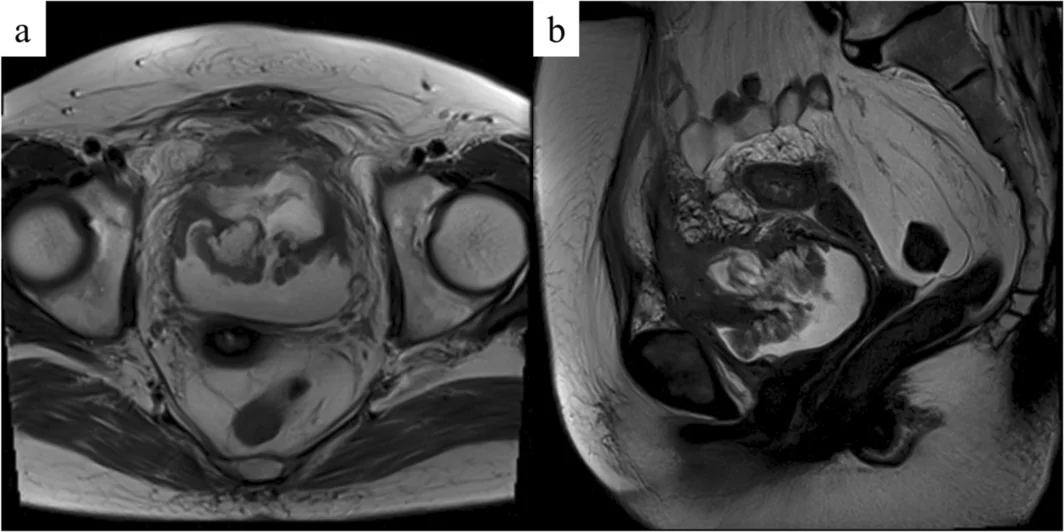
MRI findings at diagnosis. (a) Axial T2‐weighted image demonstrating a heterogeneous, predominantly hyperintense mass arising from the bladder dome, characterized by mixed solid and mucinous components. Nodular soft‐tissue lesions are present along the peritoneal surface, consistent with peritoneal dissemination. (b) Sagittal T2‐weighted image clearly showing a midline supravesical mass extending toward the urachal remnant with extravesical growth. Additional small nodules along the anterior peritoneum further support the presence of peritoneal implants.
